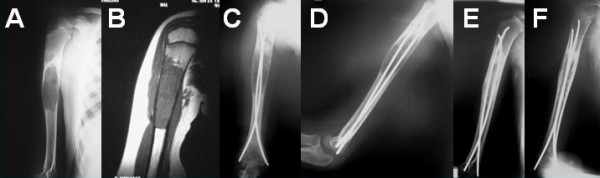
(A) Plain radiograph and (B) magnetic resonance imaging of the right humerus of a 7 year-old boy with a simple bone cyst. The patient had previous steroid injections. (C) Anteroposterior and (D) lateral radiographs after intramedullary nailing and injection of demineralized bone matrix and autologous bone marrow from the ipsilateral iliac crest. (E) Anteroposterior and (F) lateral radiographs at 31 months after the operation show complete healing of the cyst (Neer stage I).
